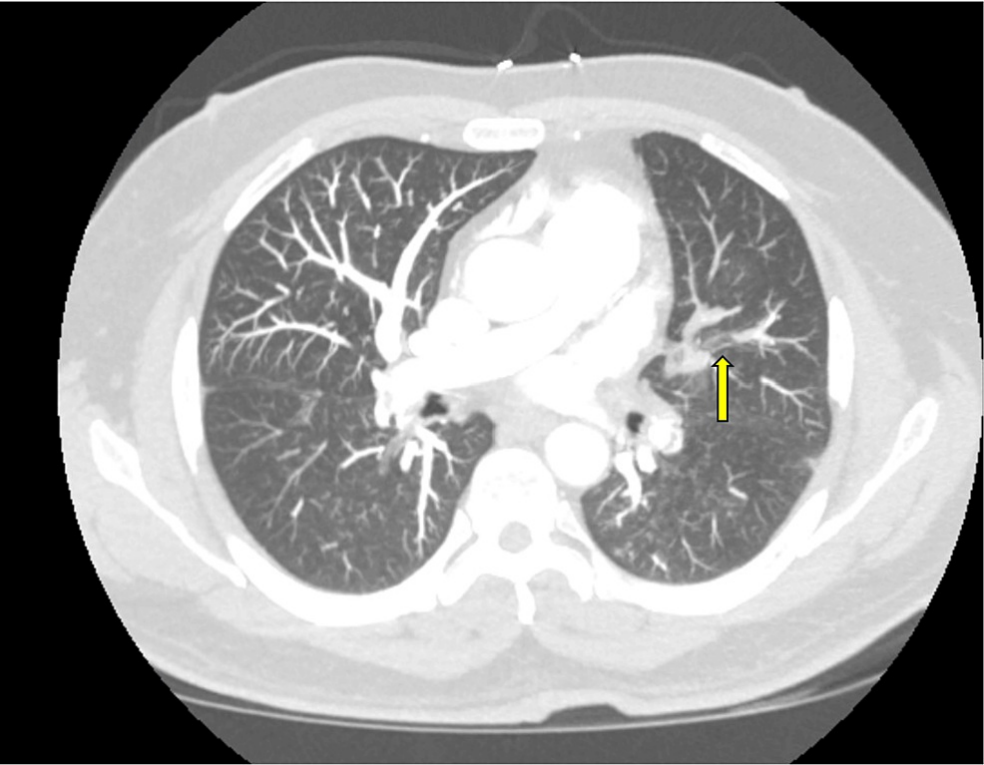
CT angiogram of the chest shows a thrombus in the left main pulmonary artery (arrow) (from last admission in 2024)
